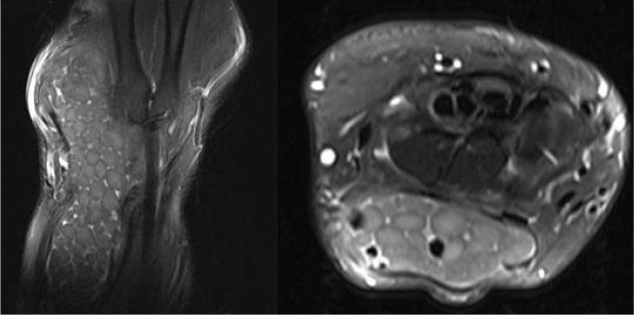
Magnetic resonance imaging of the wrist showing multiple, round, multivesicular daughter cysts occupying almost the entire volume of the mother cyst confined to the soft tissue at the extensor surface.
